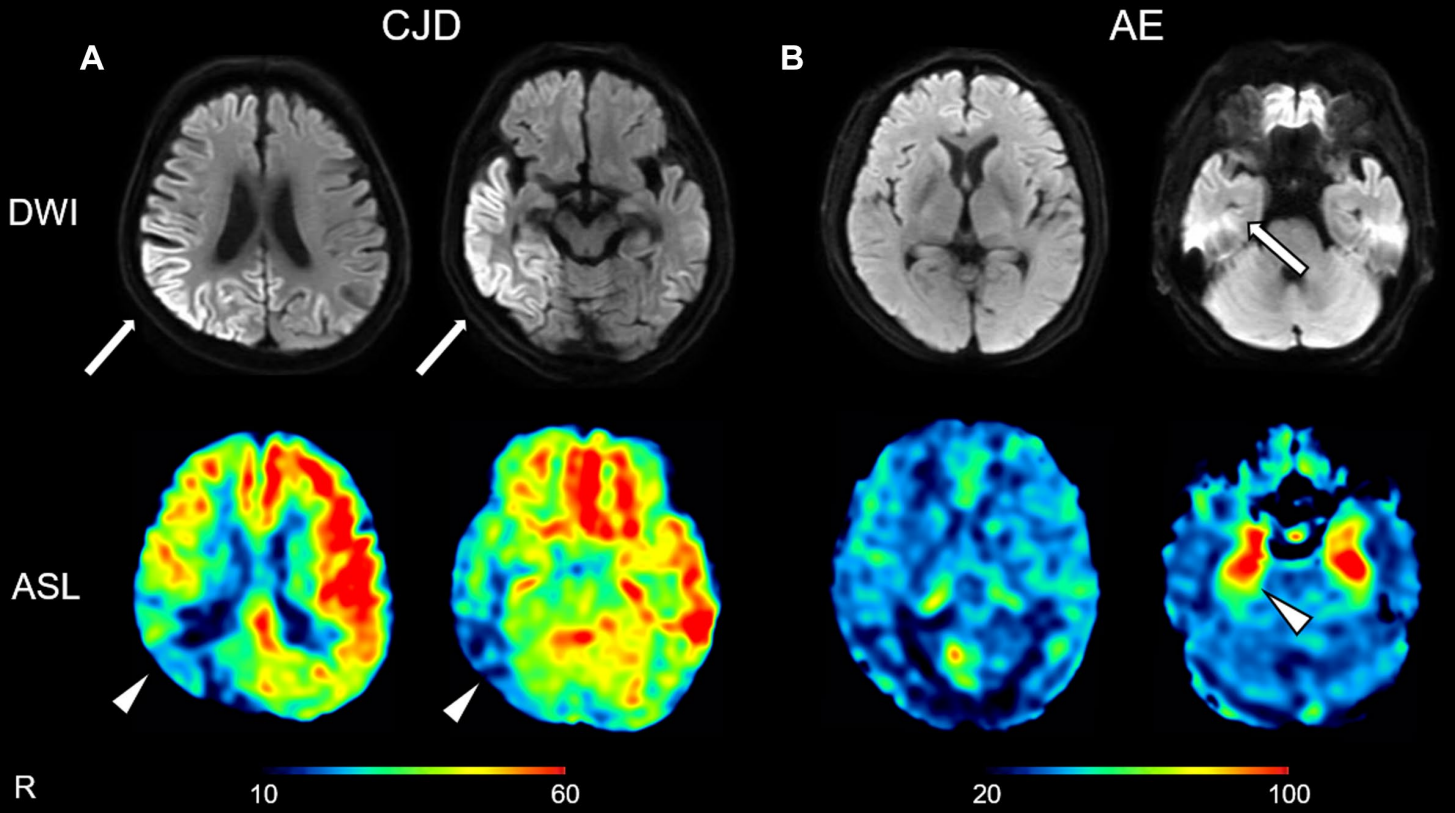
Representative diffusion-weighted images (DWIs) and cerebral blood flow maps derived from arterial spin labeling (ASL) imaging of a patient with Creutzfeldt–Jakob disease (CJD) (A: CJD 4, patient in their 70s) and a patient with autoimmune encephalitis (AE) associated with anti-leucine-rich glioma-inactivated 1 antibodies (B: AE 3, patient in their 60s). The patient with CJD exhibited DWI hyperintensity in the right parietal and temporal regions (arrows) that showed ASL hypoperfusion (arrowheads) (A). The patient with AE exhibited DWI hyperintensity in the right temporal region (arrow) that showed ASL hyperperfusion (arrowhead) (B). The color bars indicate the range of cerebral blood flow values (ml/100 mg/min) (A,B).
